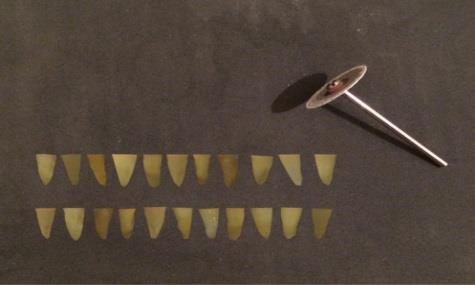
The working length of all the root canals was determined using radiography and all the root canals were filed up to #35 K-file (Maillefer) and irrigated frequently with 5.25% sodium hypochlorite solution. The MAF for all the teeth was considered file #45. Then the teeth were flared up to file #60 using the step-back technique (all the files were from the Maillefer Company and were 25mm in length)(Figure 2).
